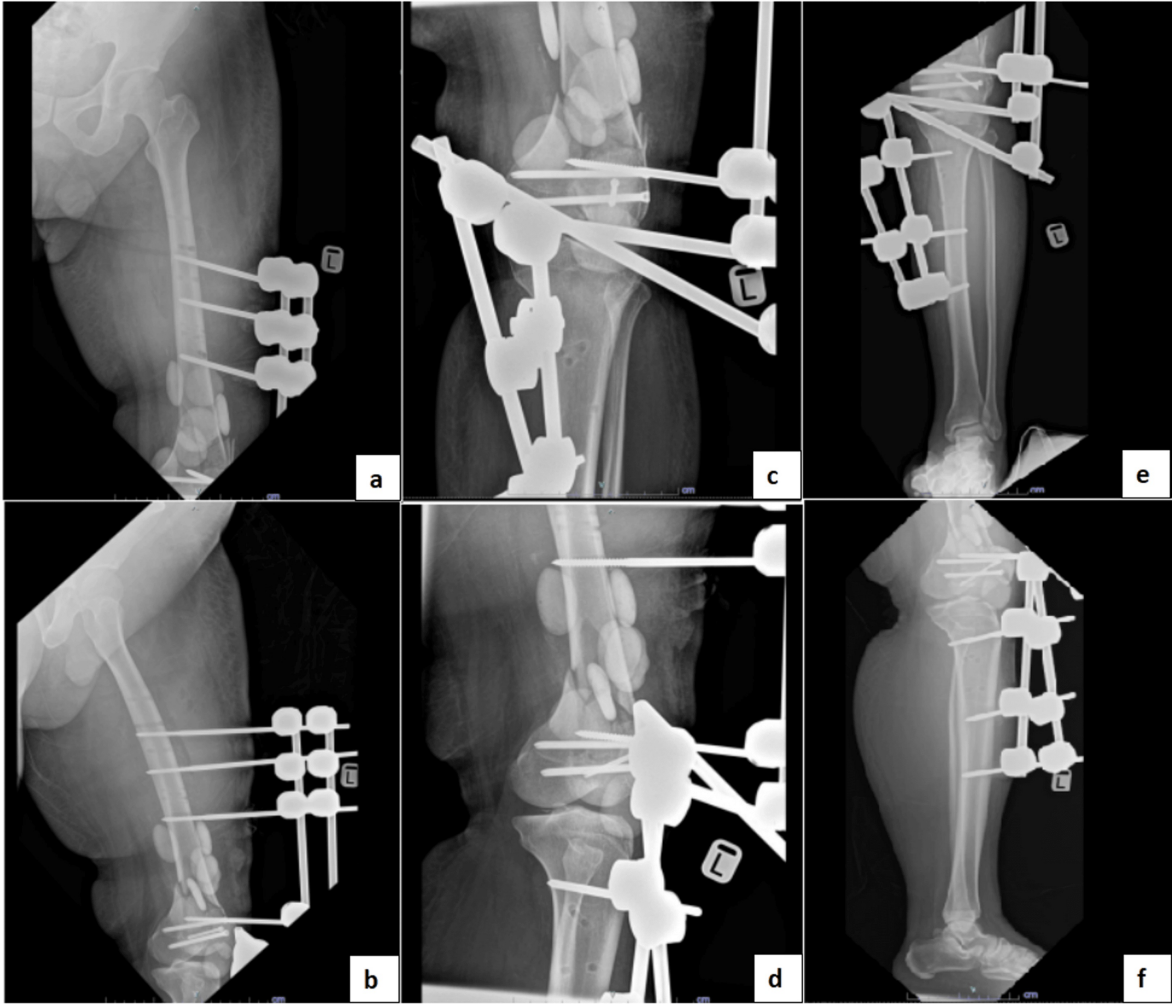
Radiographs after the readjustment of left knee cross-knee external fixator and gentamicin bead insertion. (a) Femur anteroposterior view. (b) Femur lateral view. (c) Knee anteroposterior view. (d) Knee lateral view. (e) Tibia/fibula anteroposterior view. (f) Tibia/fibula lateral view.
